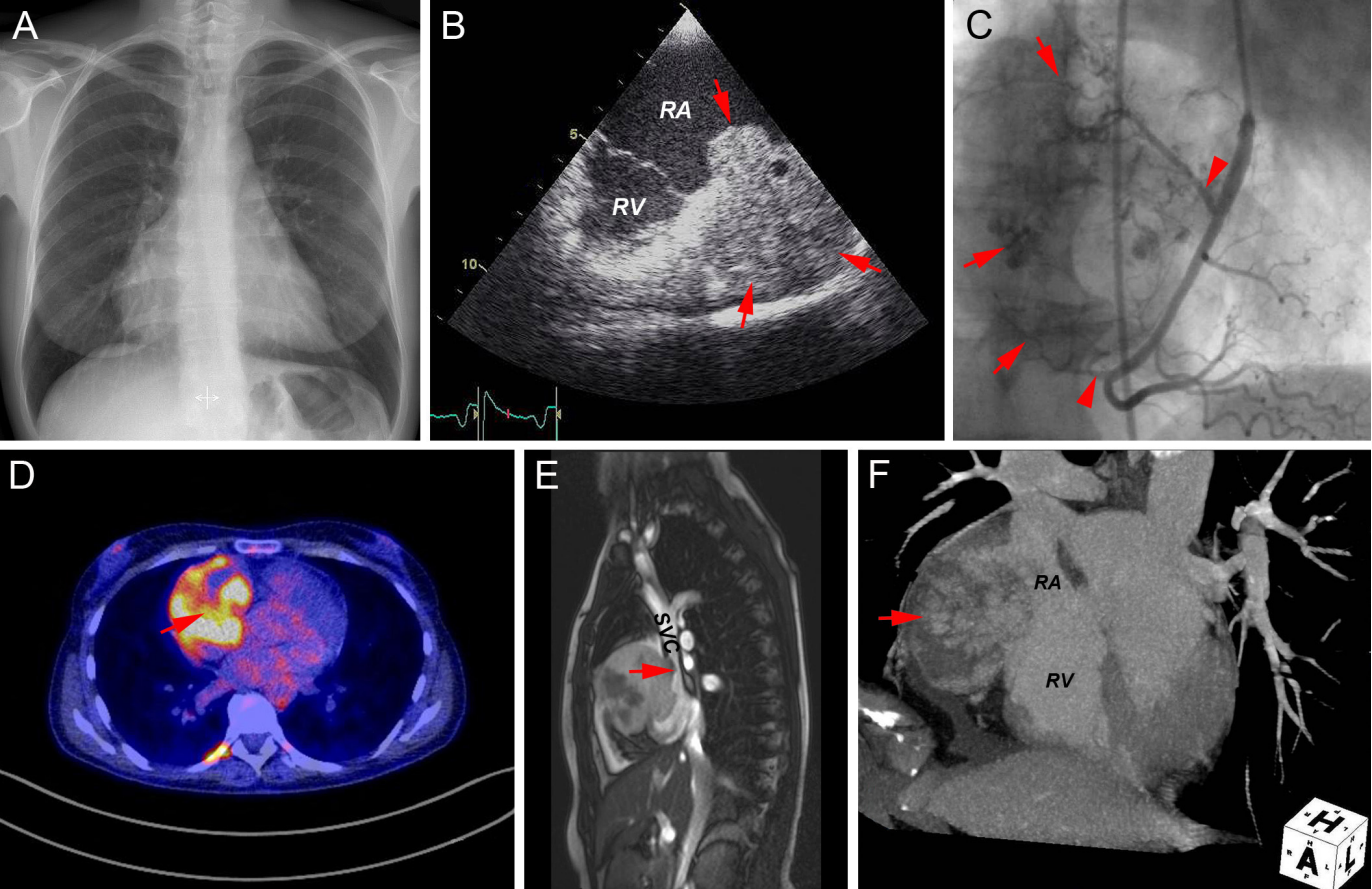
Preoperative imaging of primary right atrial angiosarcoma. A. The chest X-ray shows enlargement of the right atrial border (the cardiothoracic ratio is 0.56). B. Transthoracic echocardiography (TTE) (two chamber view of the right heart); confirms pericardial effusion, and shows a giant mass (51 × 44 mm) that infiltrates the right atrial free wall and that protrudes into the right atrium (red arrows). C. Contrast angiogram of the right coronary artery (right anterior oblique projection) showing the right coronary artery and two right atrial branches (red arrowheads) with several small areas of abnormal contrast enhancement, characteristic of a "tumor blush" (red arrows) (representing new vessel formation feeding the angiosarcoma). D. FDG (fluorodeoxy-glucose-18) PET-CT scanning (transverse section, four chamber view) to assess metabolic activity reveals hypermetabolic uptake of FDG in the right atrium (red arrow), consistent with malignancy. Paravertebrally, there is physiologic brown fat activity. E. Turboflash 2D cine MRI (sagittal section, through the superior vena cava); large inhomogenous tumor in the lateral wall of the right atrium (diameter of approximately 74 mm), extending into the wall of the superior vena cava (red arrow). F. CT (reconstruction along the cardiac axis); large inhomogenous tumor in the lateral wall of the right atrium, protruding into the right atrium (64 × 53 mm), without invasion of the right ventricular wall (red arrows). *A, coronal plane; L, sagittal plane; H, horizontal plane; RA, right atrium; RV, right ventricle; SVC, superior vena cava*.
